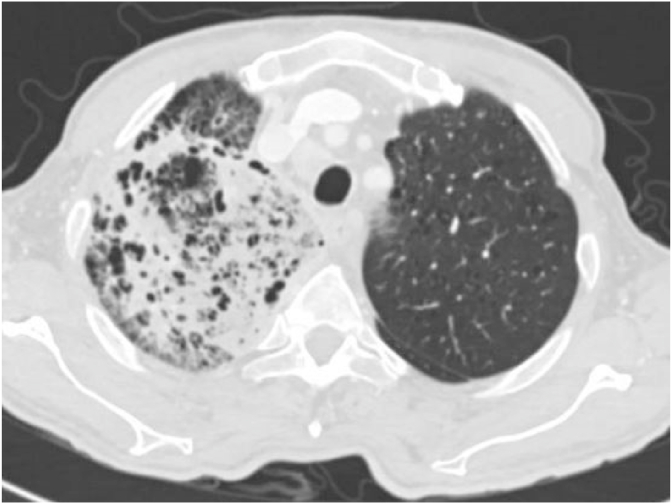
CT Chest showing extensive ground glass and cystic changes in right upper lobe.
